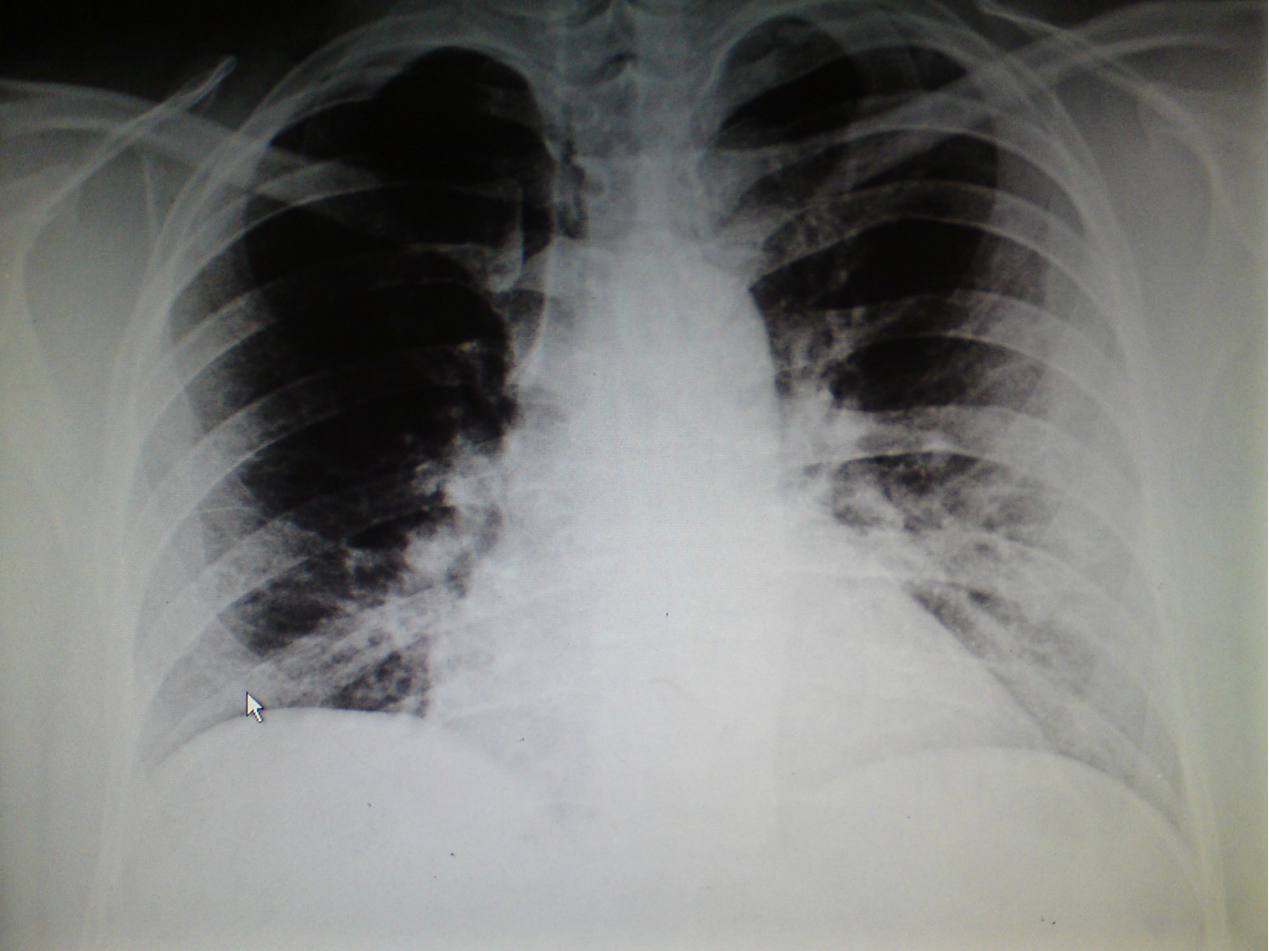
Normal erect chest x-ray. No air under the diaphragm.
